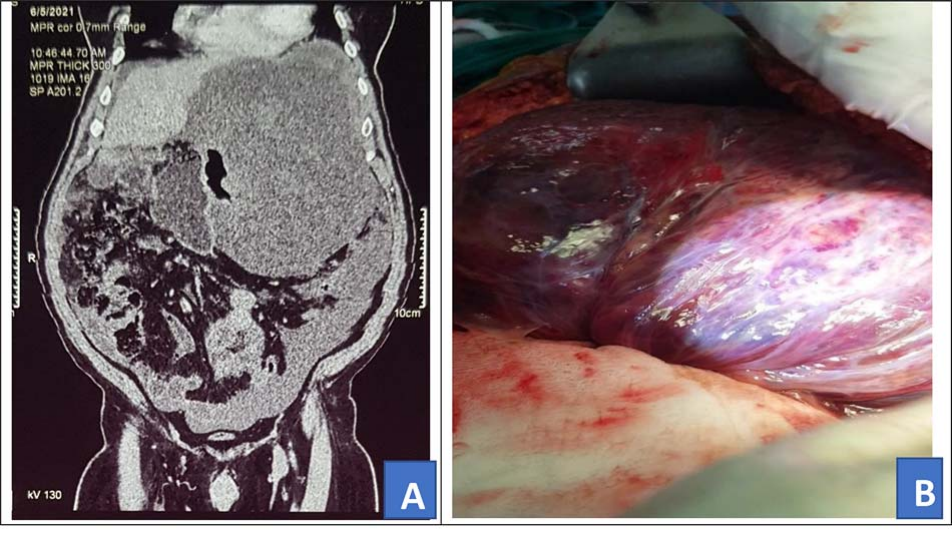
Preoperative abdominal CT scan (A) and intra-operative picture (B) for Patient 2.
